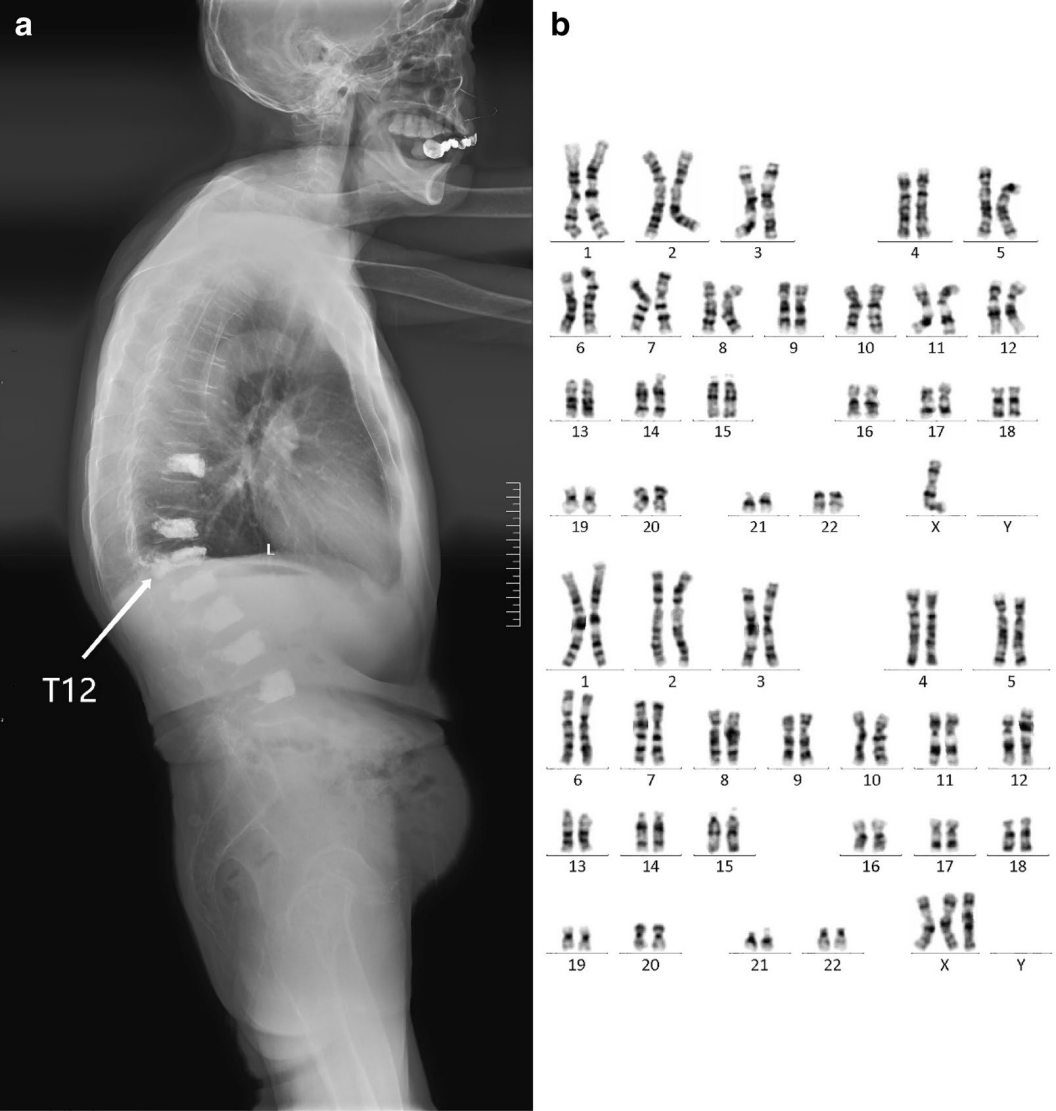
Imaging studies and karyotype analysis. a The whole spine erect lateral projection radiograph shows the patient had received seven times of PVPs at T9, T11–L4. The minimum scale of the ruler in this radiograph represents 5 mm. b The result of G-banded karyotype analysis shows the karyotype of this patient is 45,X[43]/47,XXX [17]
